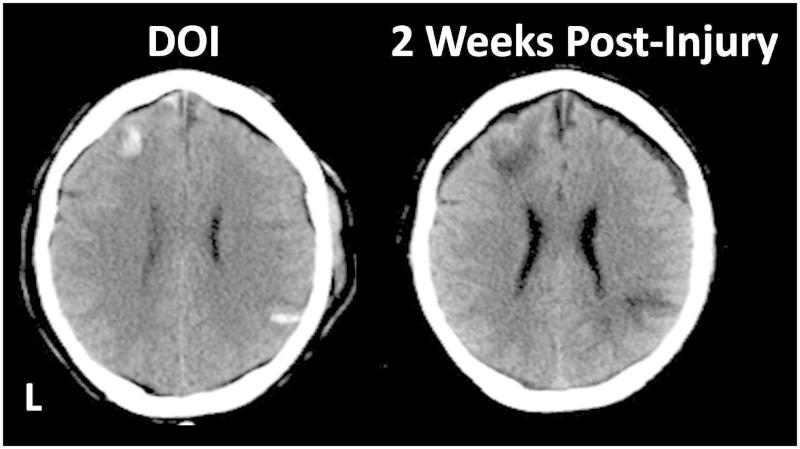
L = Left. The CT image on the left is from the day-of-injury (DOI) and shows focal intraparenchymal haemorrhage in the left frontal lobe with a contrecoup focal haemorrhagic lesion located posteriorly in the right parietal area. By 2 weeks post-injury, focal areas of decreased density deep within the frontal and parietal parenchyma have developed, reflecting damage substantially larger than the original haemorrhagic lesion.
